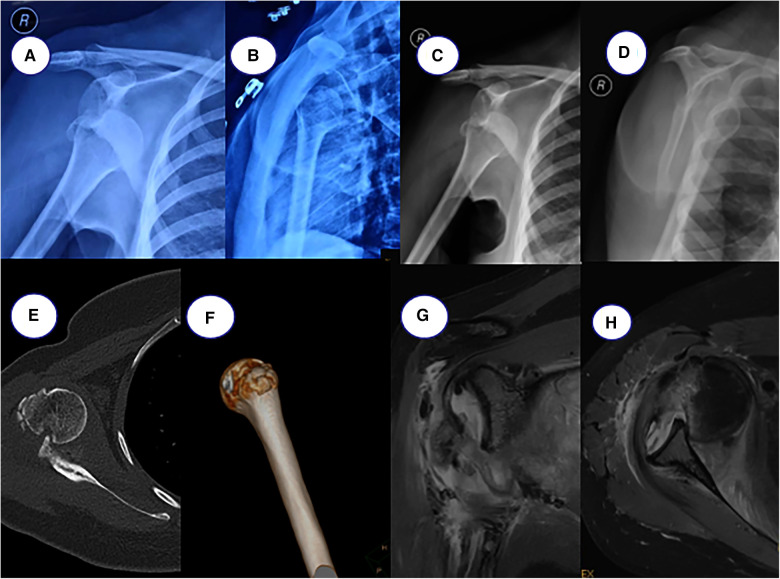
(A,B) postoperative x-ray after external emergency repositioning, (C,D) preoperative x-ray, (E,F) preoperative CT film, (G,H) preoperative MRI.
